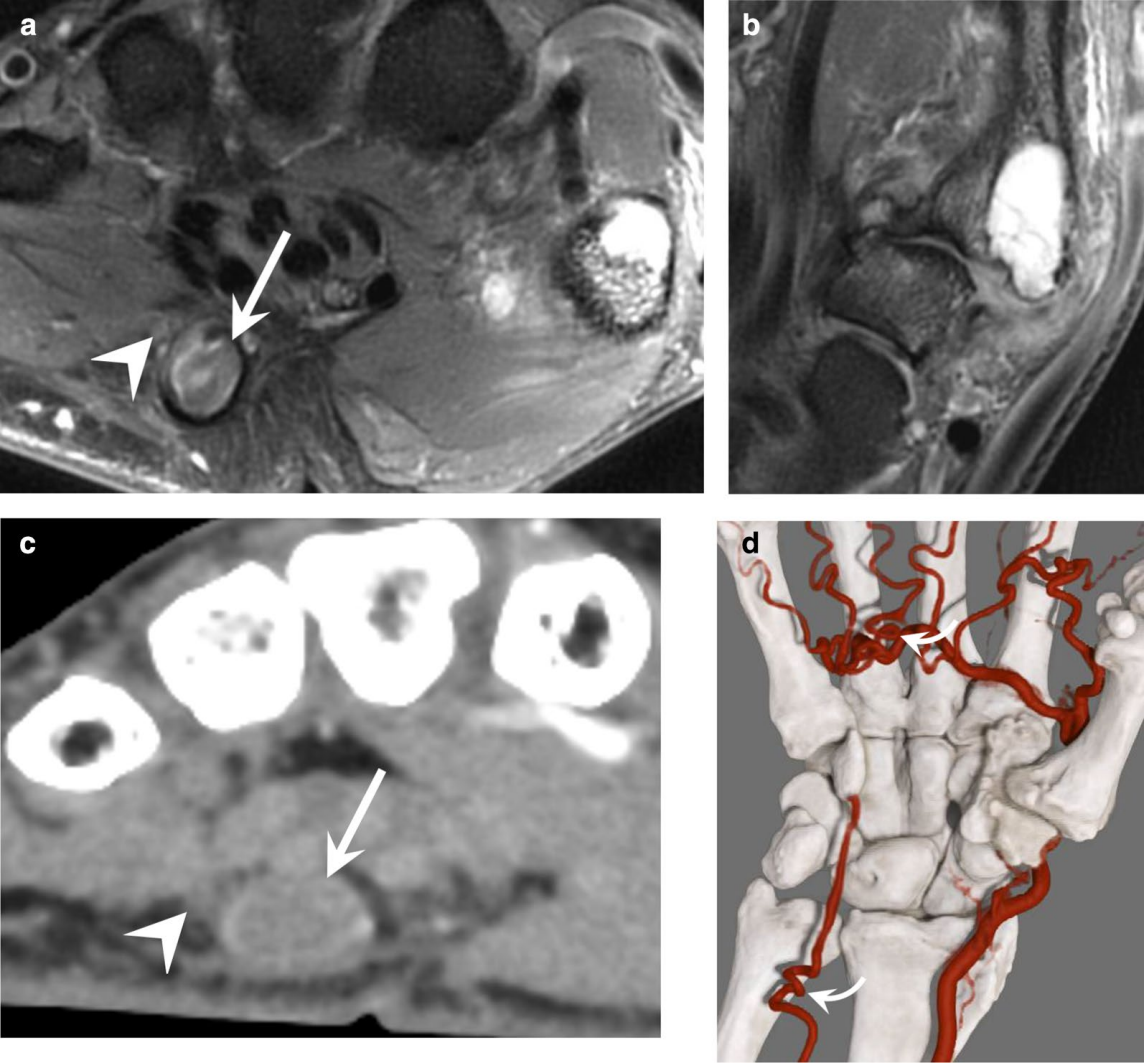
HHS in a 55-year-old manual worker exposed to different causative factors, including vibrating tools. a, b Axial and coronal T2-weighted MR images showing an occluded aneurysm (arrow) of the distal portion of the distal UA and severe osteoarthritis affecting the trapeziometacarpal joint. c, d Axial image and GI with dynamic CTA showing the distal occlusion of the UA, the patency of the SPA fed by the FPMA, a corkscrew deformity of common digital arteries, and the UA at the level of the ulnar head (curved arrow). Note the close relationship between the aneurysm and the superficial branch of the ulnar nerve (arrowhead)
